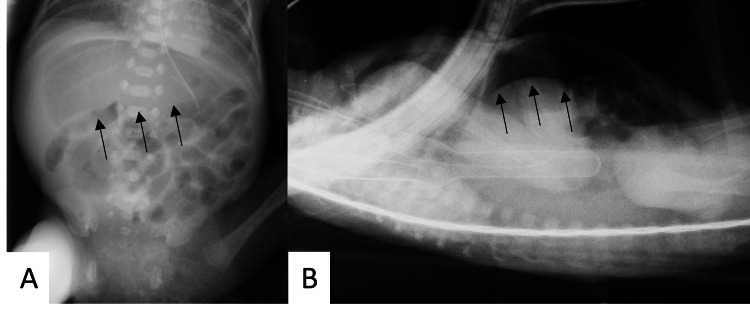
Radiographs demonstrating free intraperitoneal air (A) Supine abdominal radiograph showing free intraperitoneal air. (B) Decubitus abdominal radiograph showing free air layering within the peritoneal cavity.
